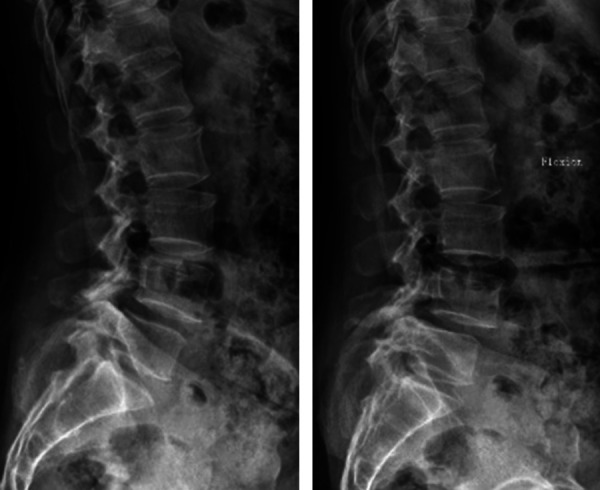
Preoperative dynamic lumbosacral radiographs showing obvious instability in the L4–L5 segment.
